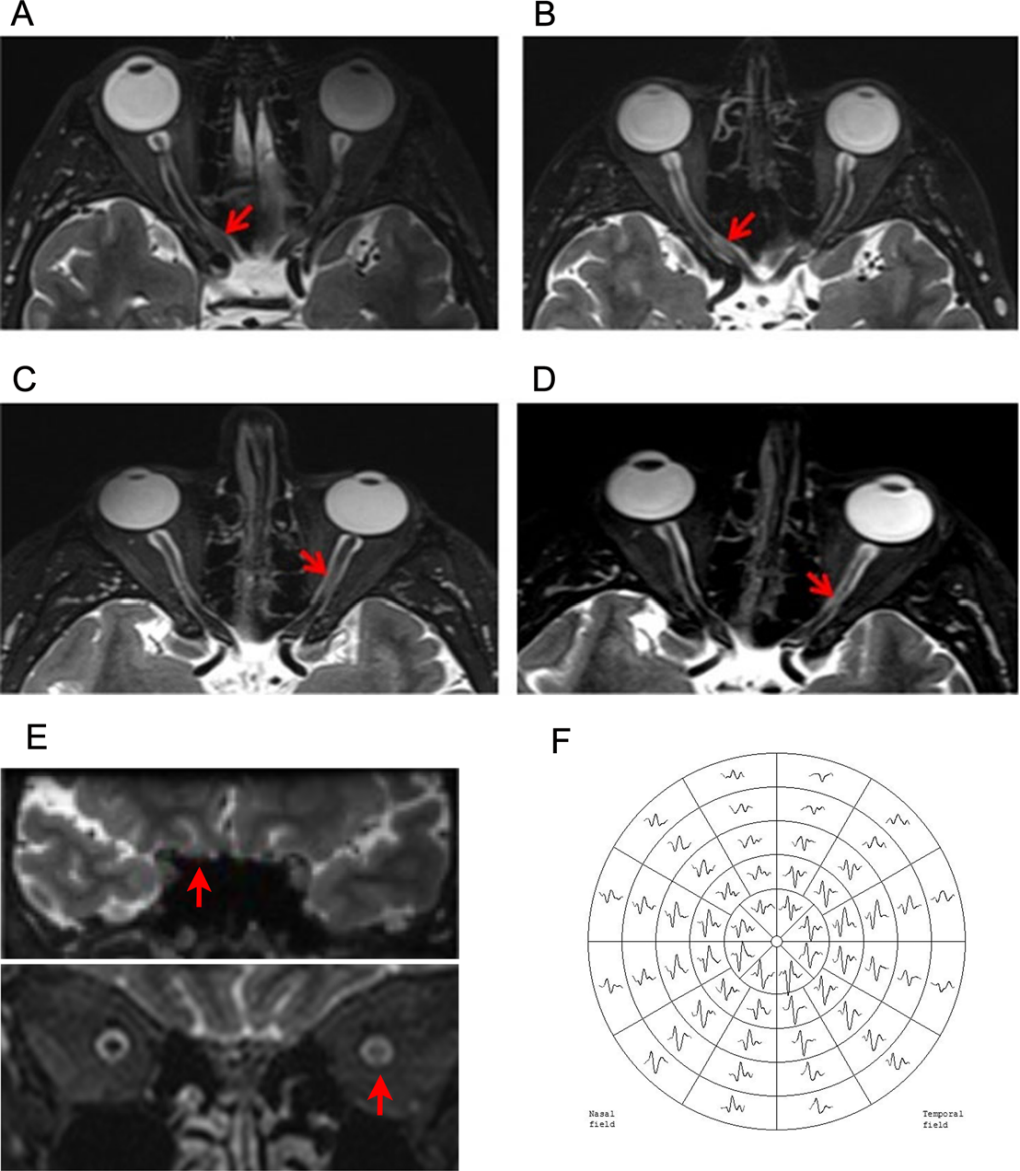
Example of optic nerve MRI lesions after ON and mfVEP recording. A-B: Intra-cannalicular lesion at 1 month (A) and 12 months (B) after ON. Lesion length decreased from 9.9 mm to 7 mm. C-D: Intra-orbital lesion at 1 month (C) and 12 months (D) after ON. Lesion length decreased from 11.3 mm to 5.5 mm. E: Coronal views of intra-cannalicular (above) and intra-orbital (below) lesions. F: Example of mfVEP trace.
